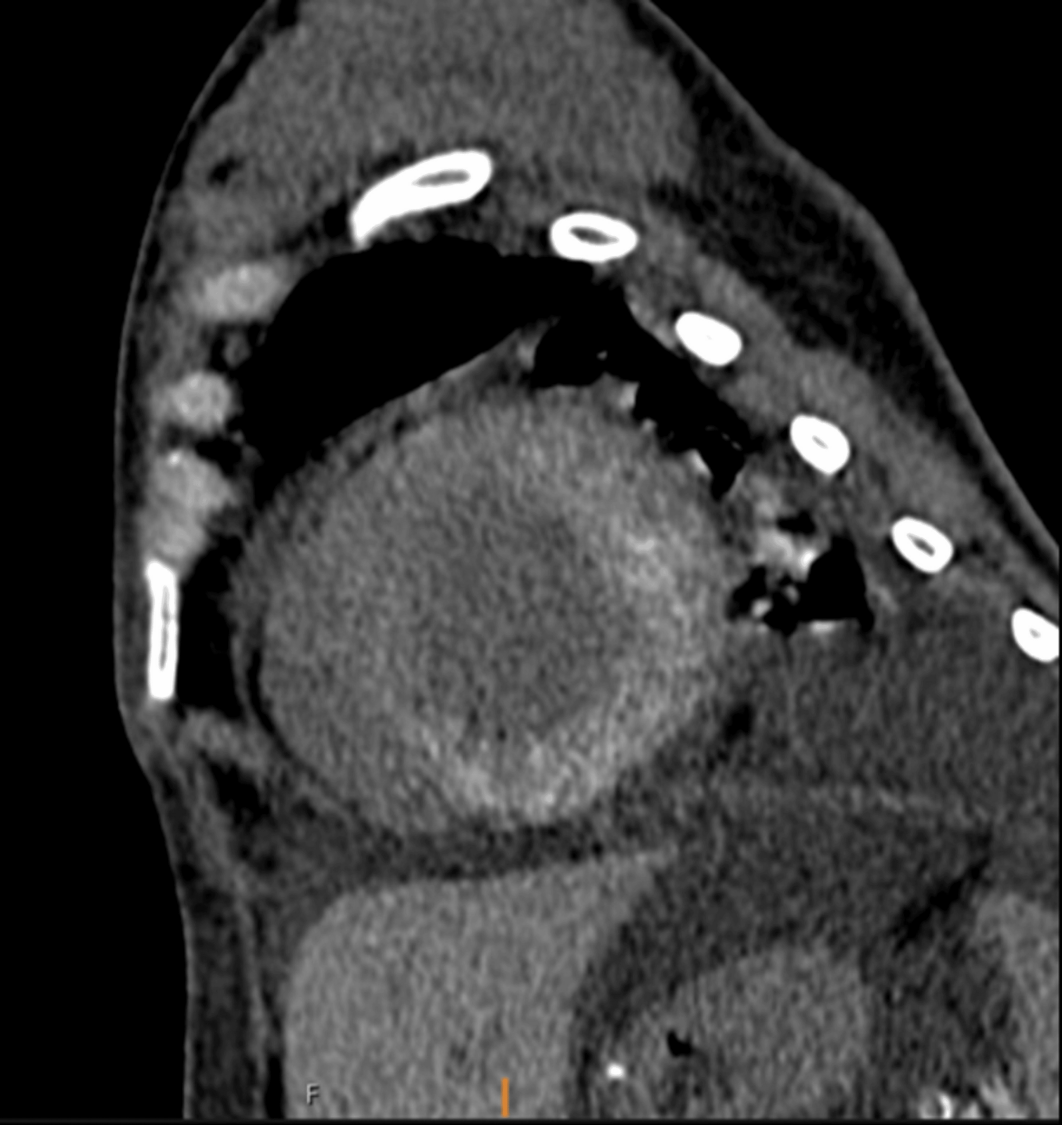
Cardiac CT with short-axis views showing diffuse hyperdense calcifications in the left ventricular myocardium. Pleural effusion is also seen.
